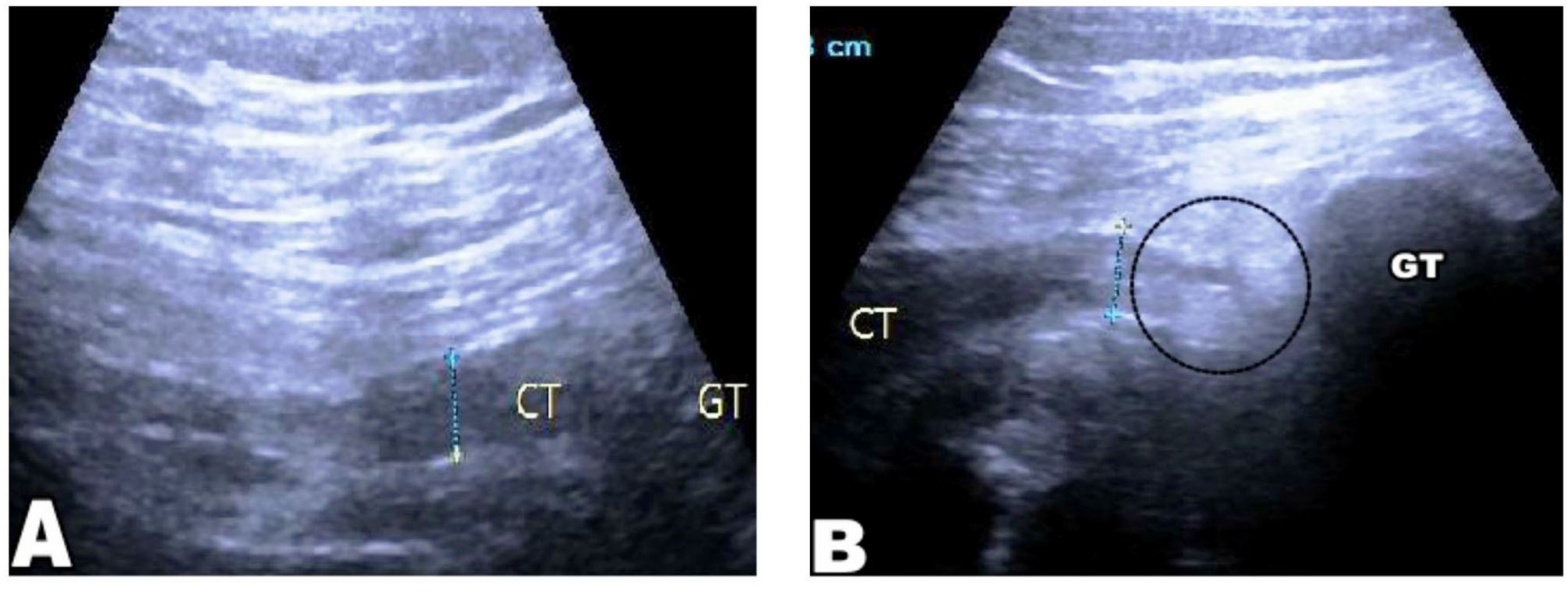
Musculoskeletal ultrasound examination, (A) “CT” indicates the preoperative conjoint tendon morphology; “GT” indicates the greater trochanter of the femur; (B) “CT” indicates the conjoint tendon morphology after lysis; the continuity of the tendon is interrupted, slightly tortuous, and has poor tension. The black circle indicates the position of the interrupted conjoint tendon.
